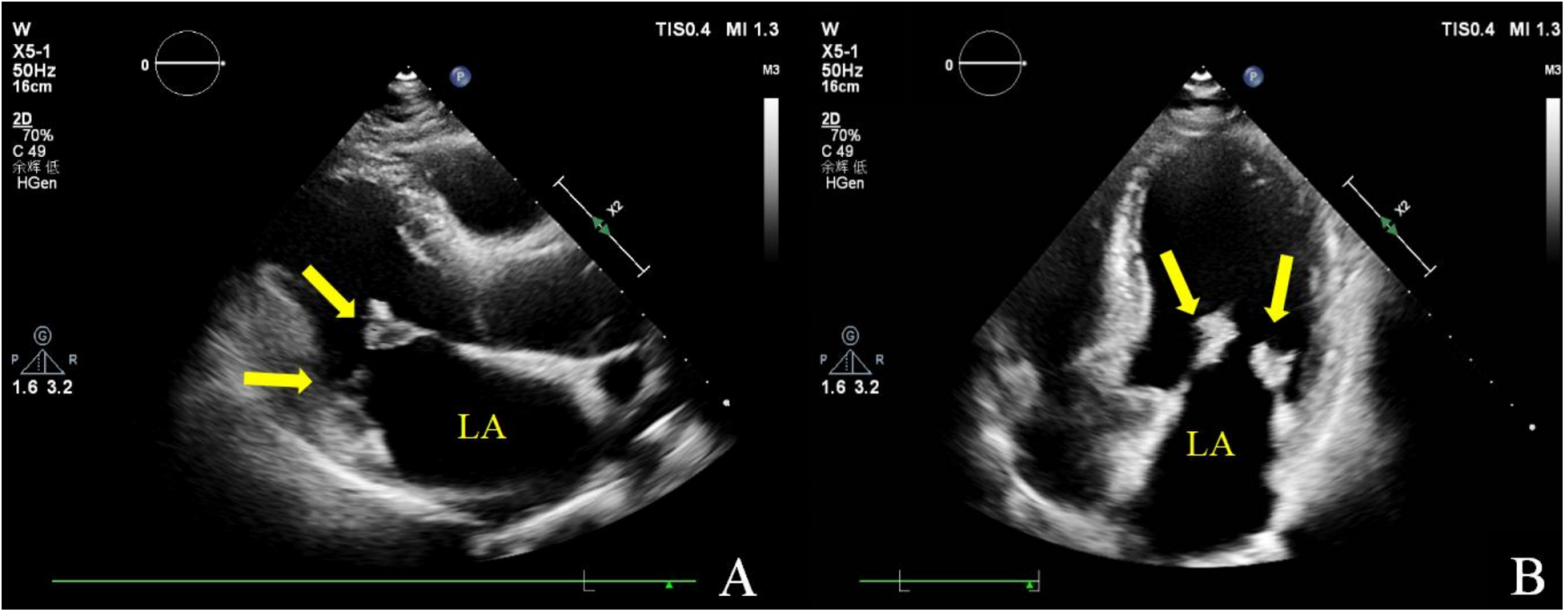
Transthoracic echocardiography. Multiple iso-echoic masses with irregular shapes were observed on the atrial sides of both the anterior and posterior mitral leaflets. Left ventricle long axis view (A) and apical four chamber view (B).
